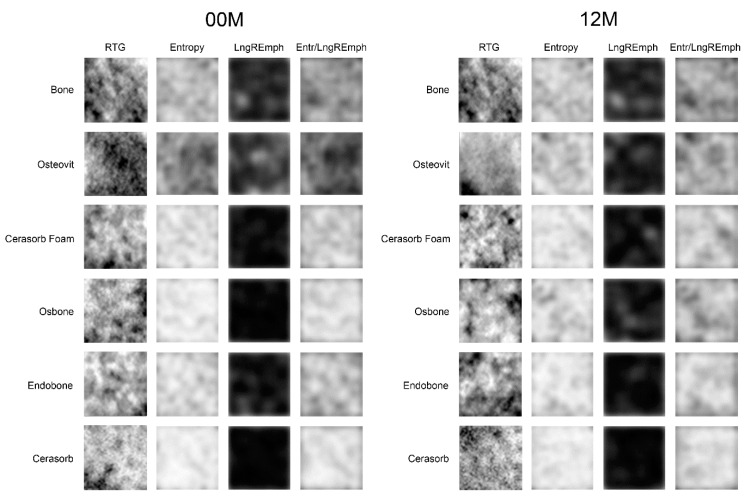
Investigated material (reference bone and five bone substitutes) and method of evaluation. Sample regions of interest in radiographs are named ROI. The following columns (Entropy, LngREmph, Entr/LngREmph) show a map of feature distribution in ROI.
